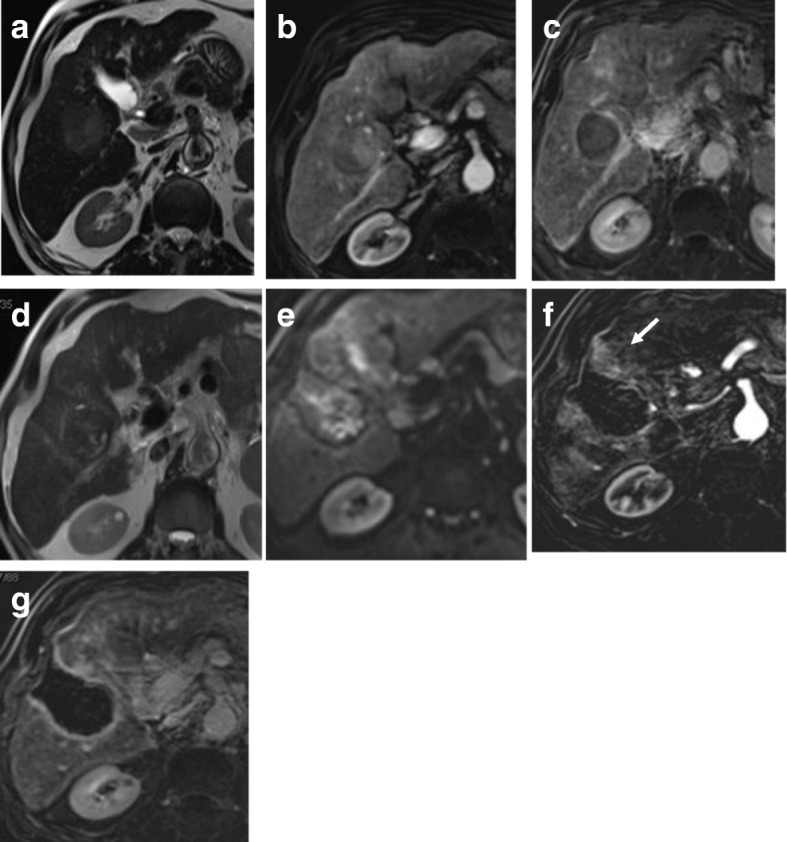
Hepatic focal lesion before (a–c) and after RFA (d–g). a T2-WI shows a well-defined HFL of an intermediate signal. b Enhanced T1-WI in the arterial phase shows early arterial heterogeneous enhancement. c Enhanced T1-WI in the delayed phase shows washout with delayed capsule enhancement. d After treatment, T2-WI shows a low signal with peripheral hyperintensity. e DWI shows central areas of restriction. f Subtracted T1-WI arterial phase shows non-enhancement of the lesions with faint peripheral enhancement (arrow). g Delayed T1-WI shows persistent peripheral enhancement. Final diagnosis is LR-TR nonviable with post-therapy changes
